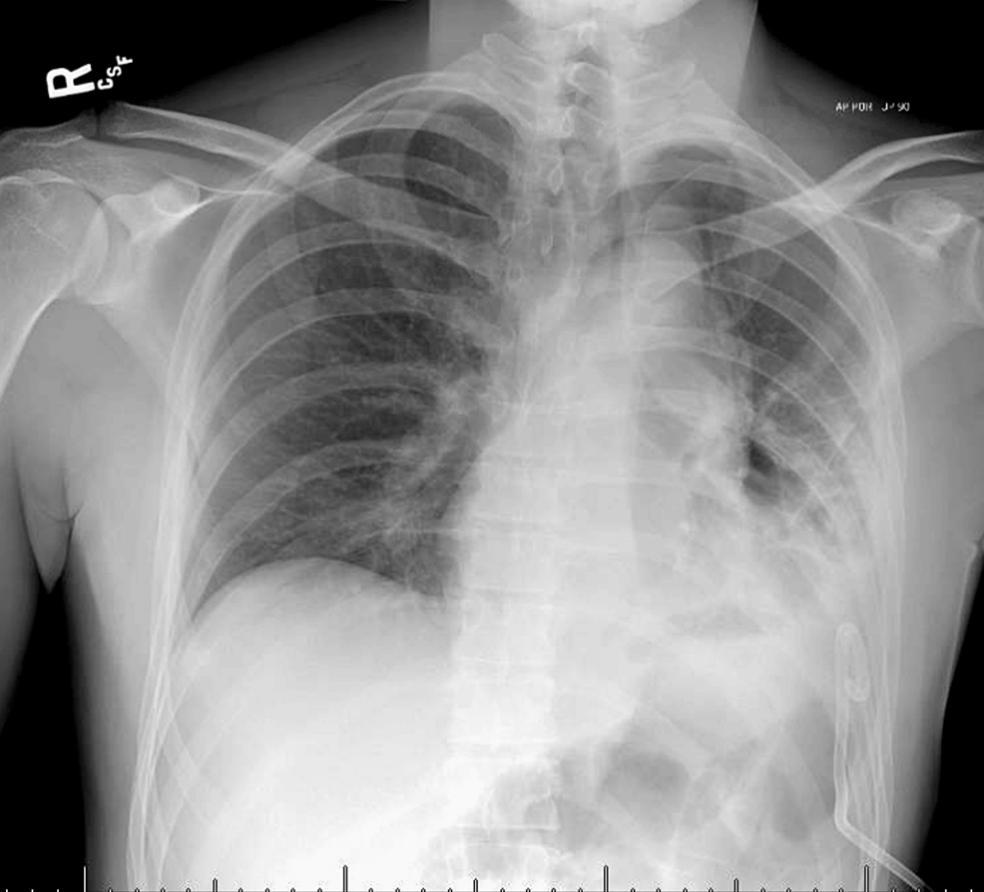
Post-chest tube placement chest radiograph demonstrating interval decrease in the left pleural effusion with a small left-sided pneumothorax.
